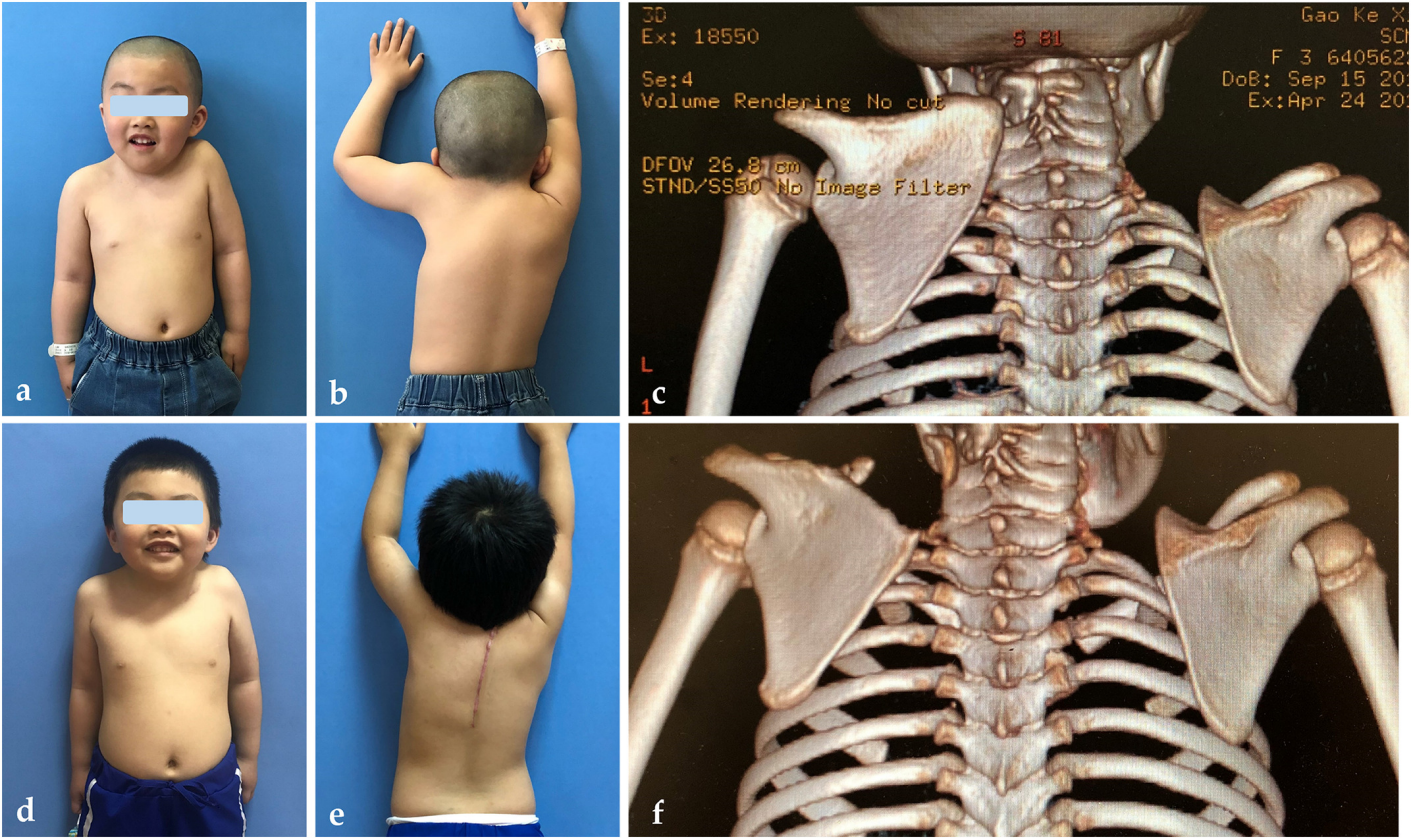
Preoperative presentation of a 4-year-and-1-month-old boy with left Sprengel deformity (Cavendish grade 4), showing appearance (a), shoulder abduction (b), and CT scan (c). The patient underwent the Woodward procedure, leading to significant postoperative improvements in appearance (d), shoulder abduction (e), and radiographic outcomes (f). Keloid formation was also observed.
